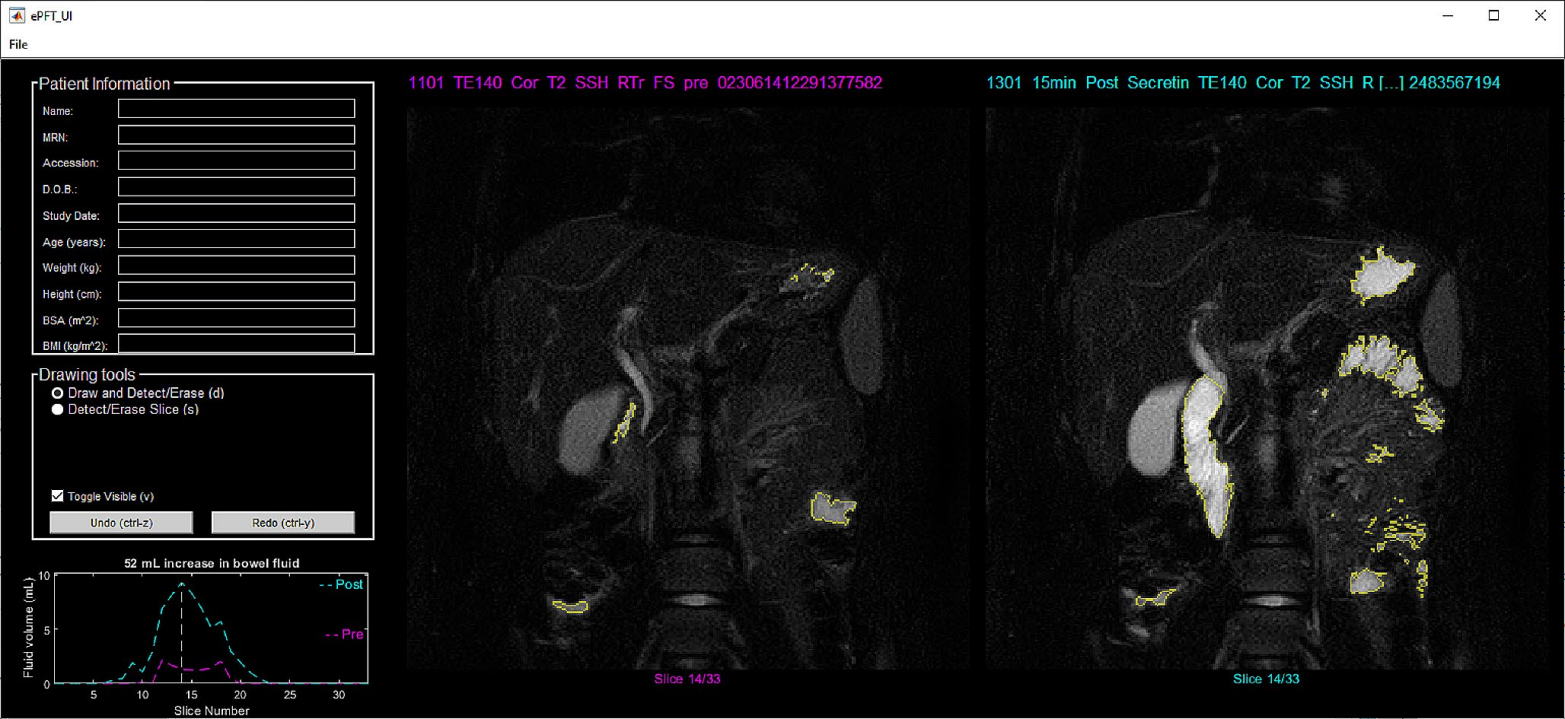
PFTquant software interface. Coronal fat-saturated images from the T2-weighted scans prior to (left image) and 15 min following (right image) secretin administration with segmented bowel fluid outlined in yellow. The upper left panel is for the display of patient information which has been redacted here. The middle-left panel contains tools for the user to edit segmentations. The lower left panel plots the pre- and post-secretin bowel fluid volumes of each slice as a fluid volume curve with the volume difference reflecting the secretory response
